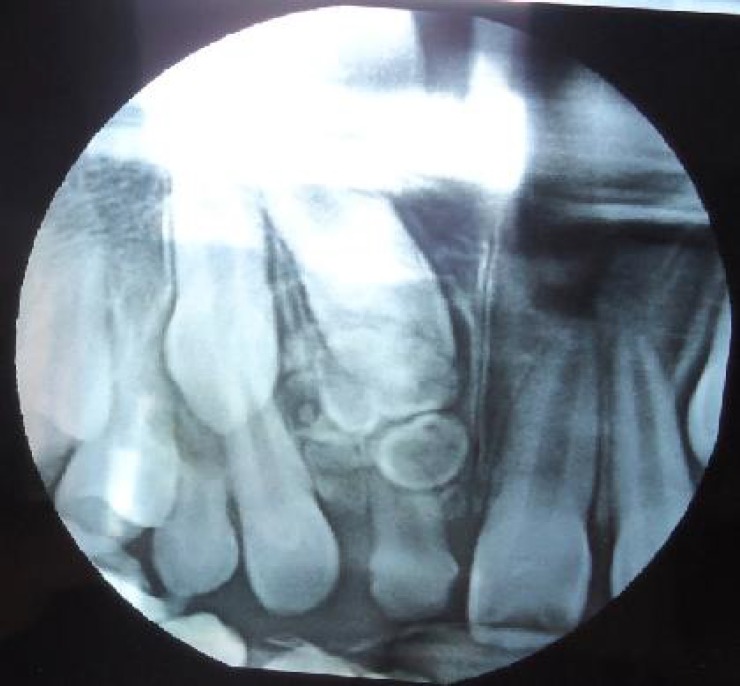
Radiograph showing presence of multiple radiopaque masses and retained permanent incisor
